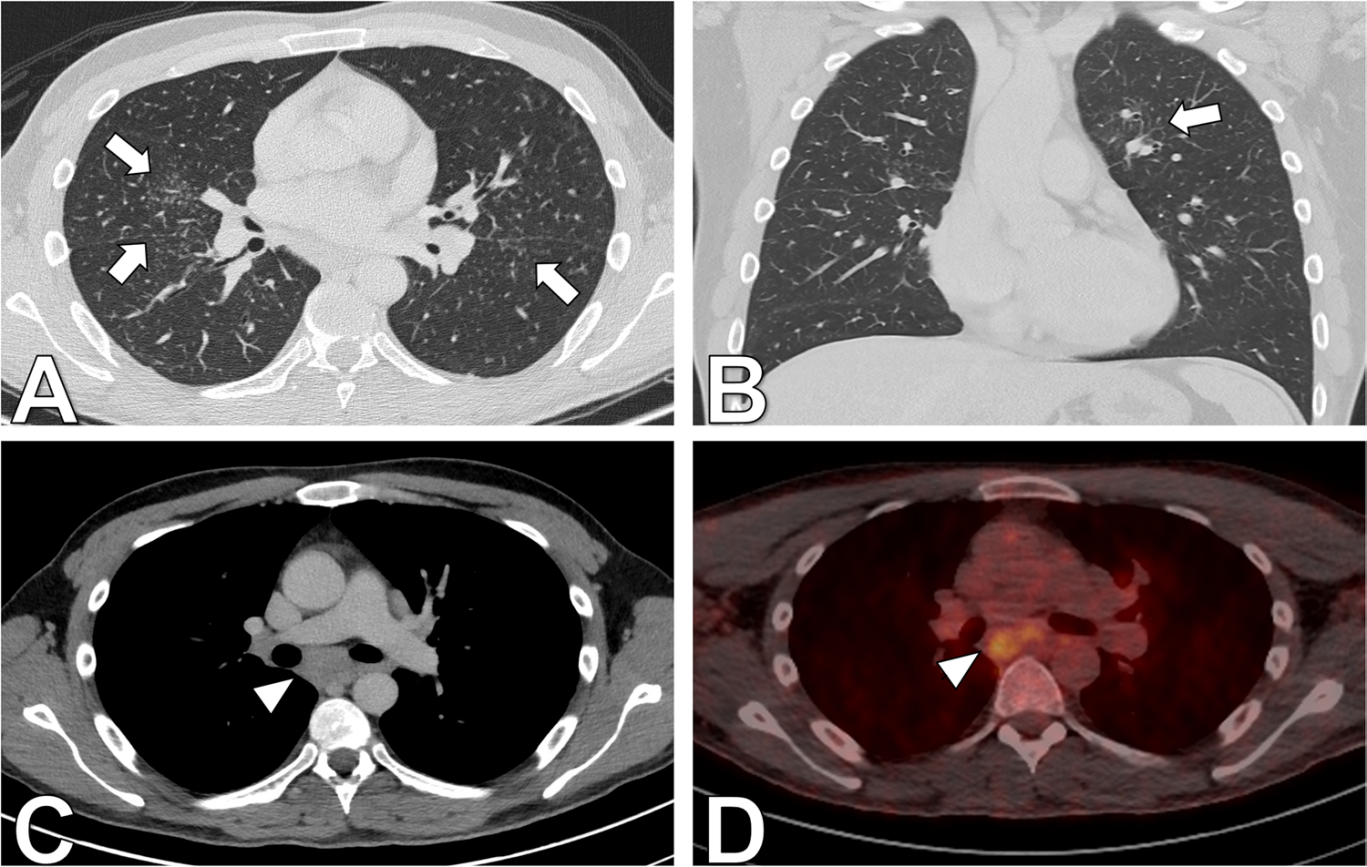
Contrast-enhanced 18-F-fluorodeoxyglucose positron emission computed tomography (PET/CT) with axial (A) and coronal (B) lung window showing fine reticulonodular opacities (arrows) of the lung parenchyma. Axial (C) soft tissue window of the mediastinum with enlarged mediastinal lymph nodes (arrowhead) and axial fused PET/CT of the mediastinum (D) with increased FDG uptake of enlarged mediastinal lymph nodes (arrowhead)
